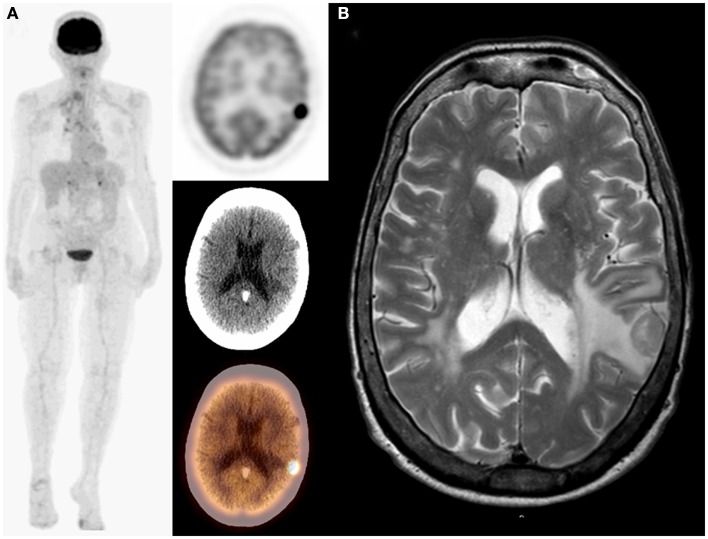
Seventy-year-old male with bronchogenic carcinoma in the right lung. On initial staging PET/CT: MIP, transaxial PET, CT, and fused images (A) demonstrated a single lesion in the right lung and unsuspected photopenia in the left frontoparietal lobe that corresponds to a hypodensity on CT suspicious for metastasis. PET/CT finding was confirmed on MRI (B). Adding the brain to the imaged field of view changed both staging (I–IV) and management as patient underwent whole brain radiation.
